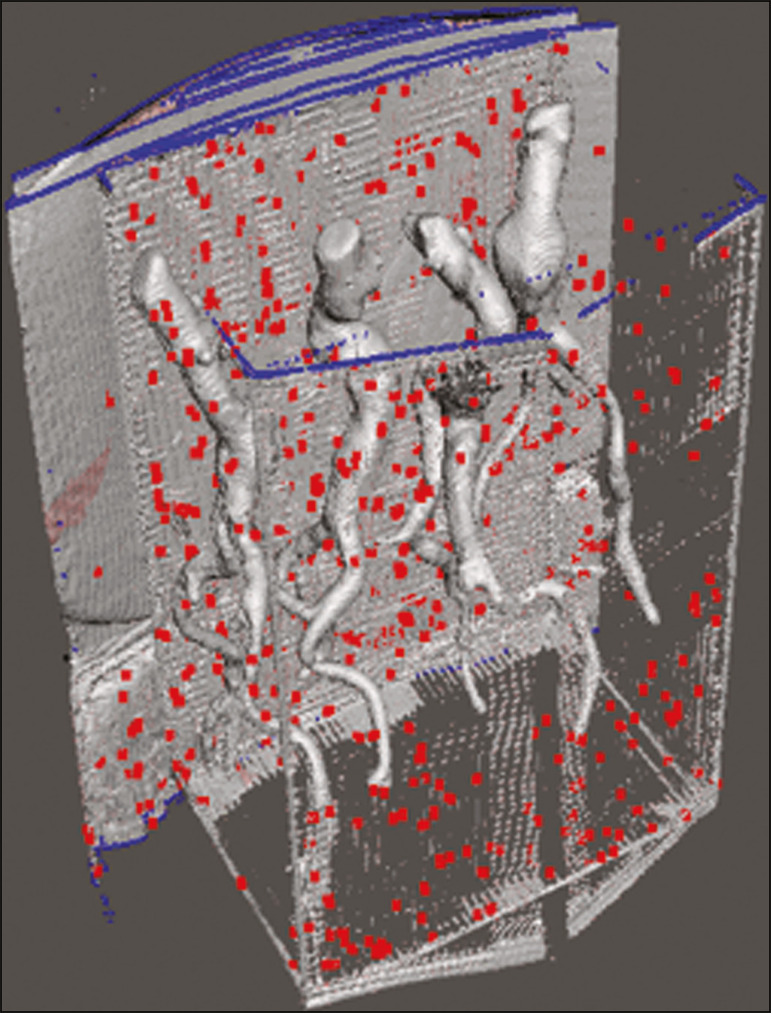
CT before deletion of the image of the cardboard box by the Meshmixer software.
